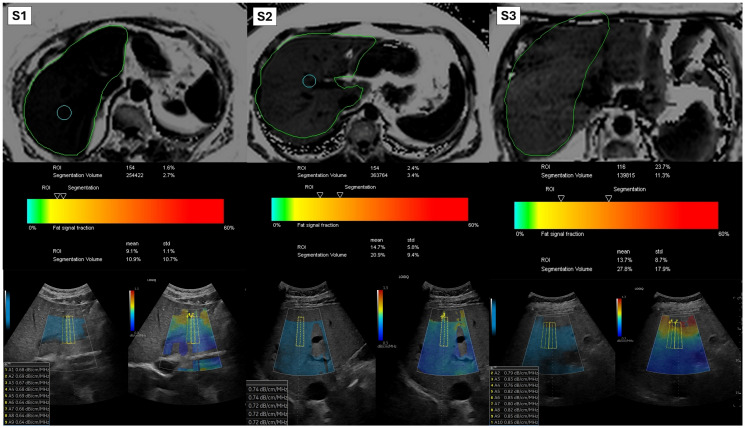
Top row: Axial MRI-PDFF maps demonstrating whole-liver segmentation and region-of-interest placement with increasing hepatic fat fraction across representative cases (S1, S2, and S3 correspond to mild, moderate, and severe hepatic steatosis, respectively). Bottom row: Corresponding UGAP acquisitions obtained from hepatic regions with quantitative attenuation maps and color scales expressed in dB/cm/MHz.
